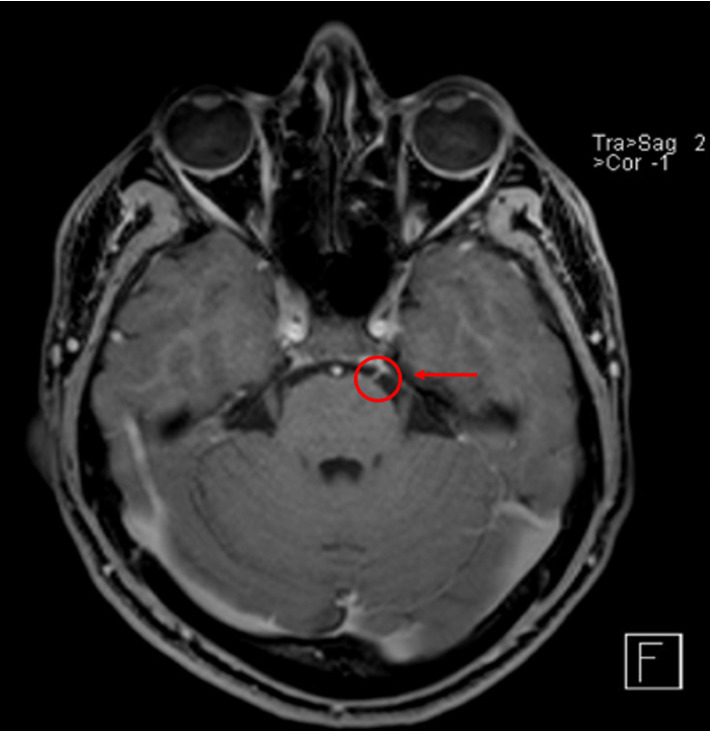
Brain MRI showing thickening and enhancement of the intracisternal segment of the left abducens nerve (red circle and arrow).
